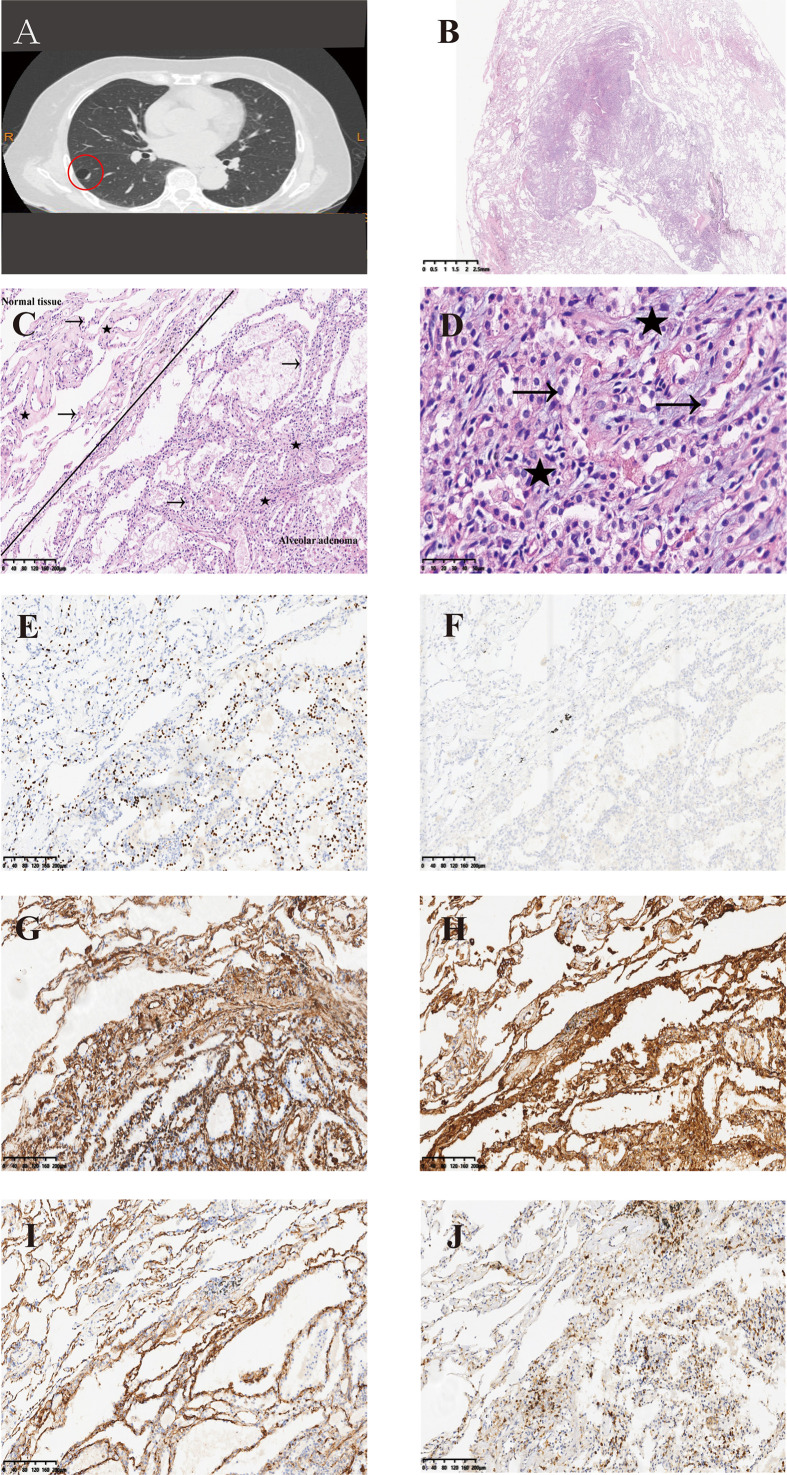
(A) CT scan displays a well-circumscribed thin-walled cystic nodule. (B) Low magnification shows the tumor with a clear boundary (10x). (C) The boundary between tumor tissue and normal tissue is clear. The tumor tissue contains well-differentiated glands (arrows), some of which are cystic, containing eosinophilic secretions, the stroma (asterisks) is loose with spindle-shaped cells exhibiting mild morphology within a myxoid matrix, along with a small number of inflammatory cells (100x). (D)Both epithelial (arrows) and stromal cells (asterisks) appear mild in morphology without notable atypia (400x). (E) Expression of TTF-1 (100x). (F) Expression of P40 (100x). (G) Expression of Vimentin (100x). (H) Expression of CD10 (100x). (I) Expression of CD34 (100x). (J) Expression of BCL-2 (100x).
